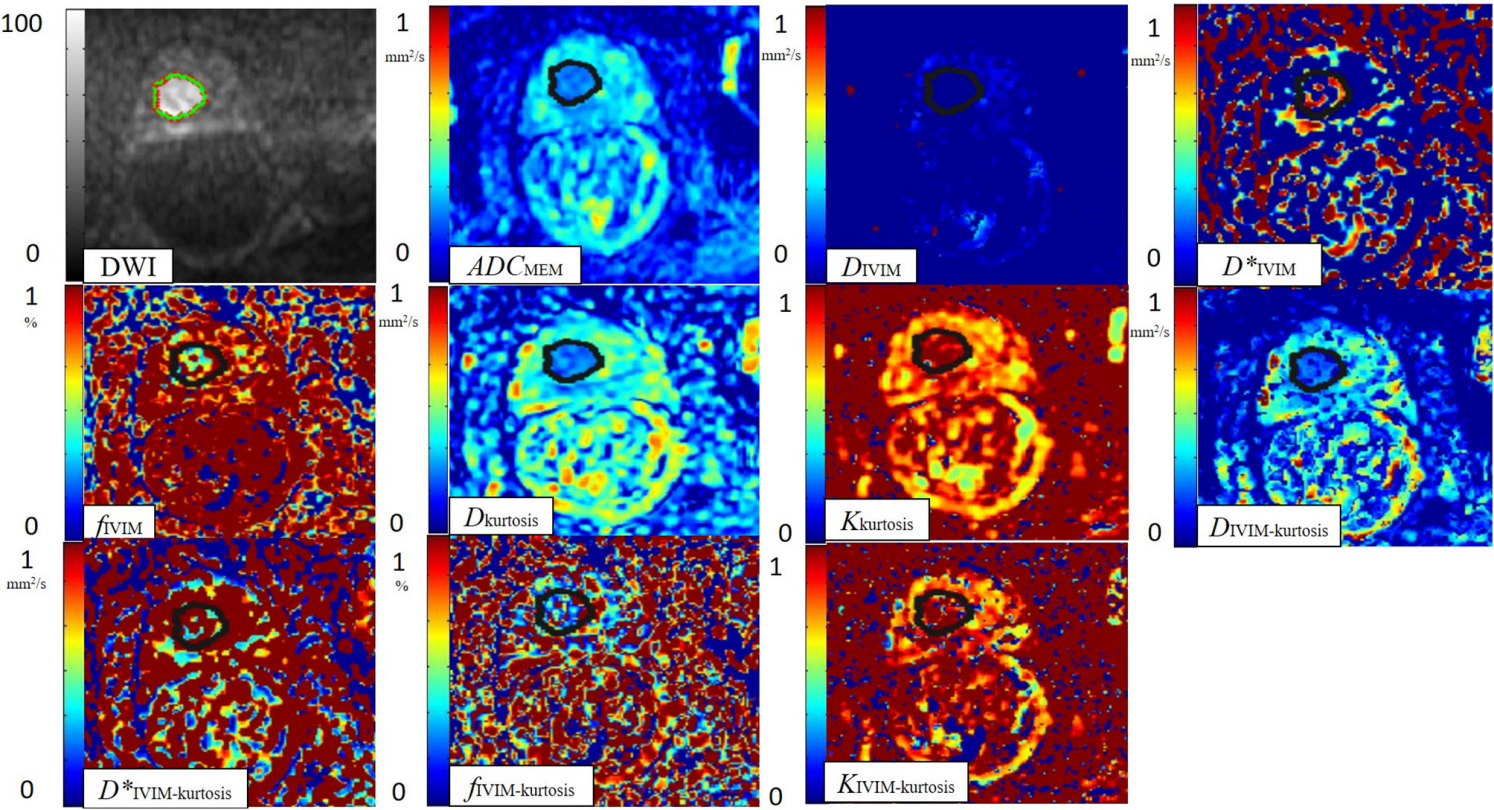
One case with PCa. A 76-year-old man with PCa (pathological GS, 3 + 4). ROI was set in PCa on DWI. ADC_MEM_, *D*_IVIM_, *D_IVIM_, *f*_IVIM_, *D*_kurtosis_, *K*_kurtosis_, D_IVIM−kurtosis_, *D_IVIM−kurtosis_, (j) *f*_IVIM−kurtosis_, and *K*_IVIM−kurtosis_ value maps. The cancer tissue showed different signals notably from normal tissues in the DWI, ADC_MEM_ (0.79 × 10^−3^ mm^2^/s), *D*_IVIM_ (0.39 × 10^−3^ mm^2^/s), *f*_IVIM_ (48.45%), *D*_kurtosis_ (1.24 × 10^−3^ mm^2^/s), *K*_kurtosis_ (0.99), *D*_IVIM−kurtosis_ (0.98 × 10^−3^ mm^2^/s), and *K*_IVIM−kurtosis_ (1.36) value maps. The PCa tissue seemed to be similar to normal tissues on the *D_IVIM_ (5.53 × 10^−3^ mm^2^/s), *f*_IVIM−kurtosis_ (24.09%), and *D_IVIM−kurtosis_ (0.78 mm^2^/s) value maps.
